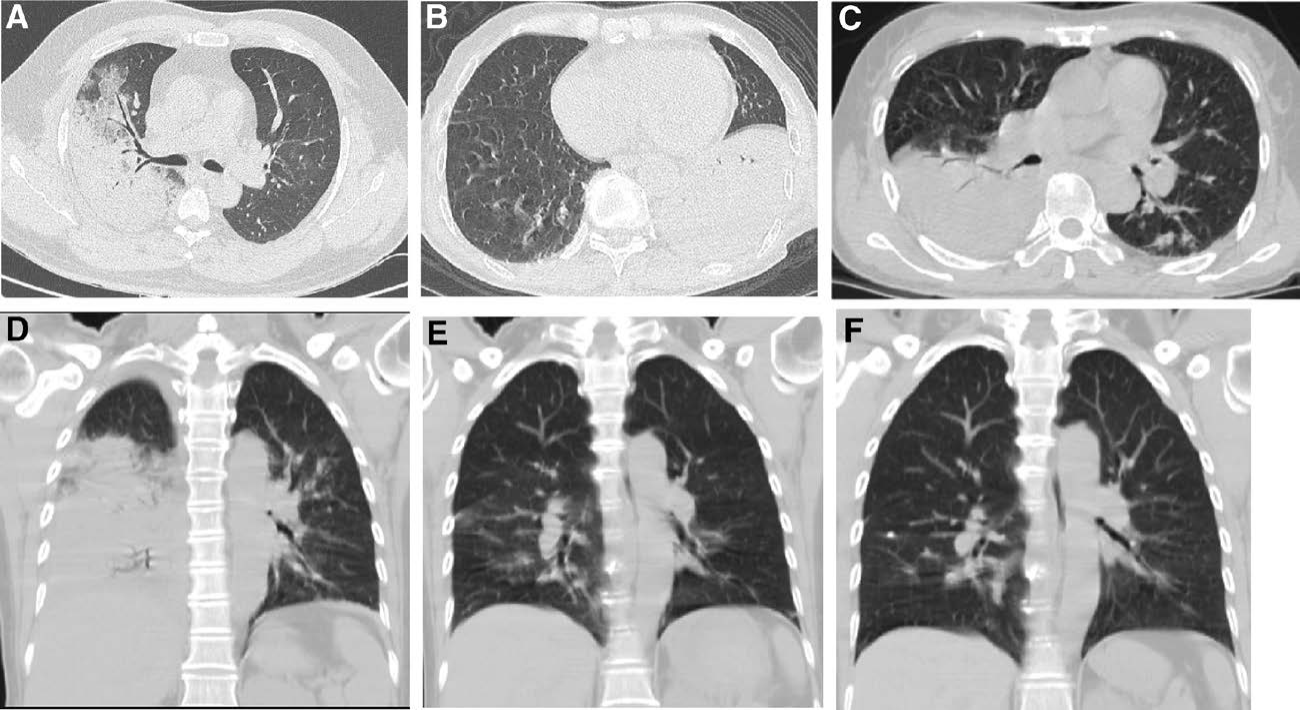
Chest computed tomography (CT) findings of severe *Chlamydia psittaci* pneumonia. (A) A 52-year-old male presenting a fever for 3 days, large air-space consolidation and air bronchogram in the right upper lobe. (B) A 47-year-old male presenting a fever for 5 days, large air-space consolidation in the left lower lobe. (C) A 48-year-old male presenting a fever for 4 days, large air-space consolidation and multi-lobe ground-glass opacity in the bilateral lung. (D–F) A 58-year-old female with the initial CT scan performed 4 days after onset showed large air-space consolidation in the right lung (D). The lesion evidently shrank and became dense following treatment with moxifloxacin and doxycycline for 7 days (E). A follow-up CT scan performed 18 days after the onset did not show any consolidation (F).
